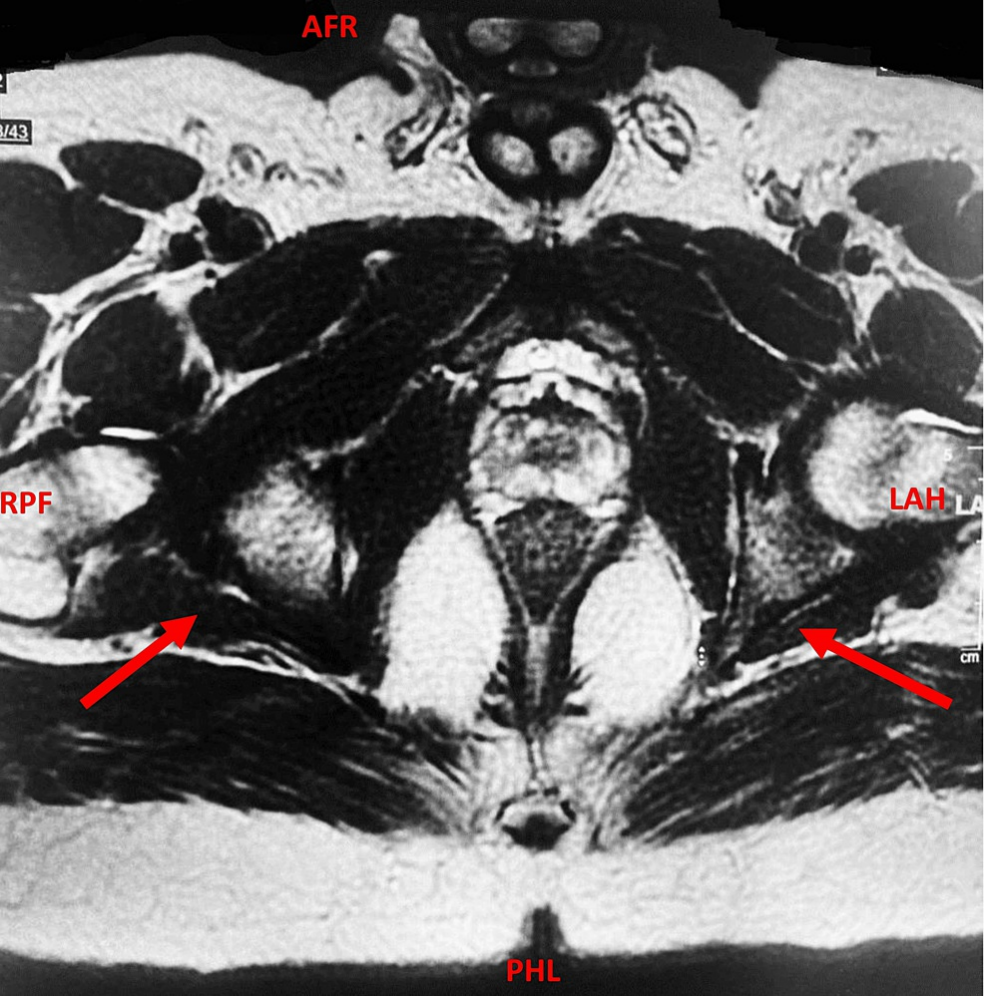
MRI scan of pelvis with IV contrast using a fistula protocol The red arrows indicate an abnormal bilateral perianal inter-sphincteric fistula with internal openings. MRI, magnetic resonance imaging; AFR, anterior-foot-right; PHL, posterior-head-left; RPF, right-posterior-foot; LAH, left-anterior-head
